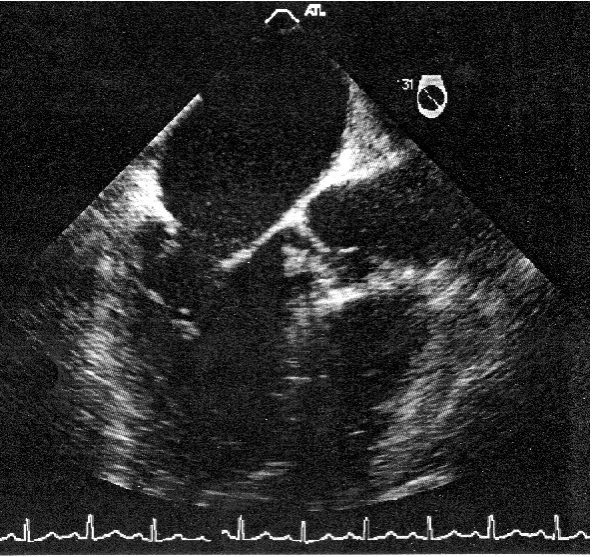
Transoesophageal echocardiography image showing a vegetation on the right coronary cusp of the aortic valve prolapsing during diastole.
